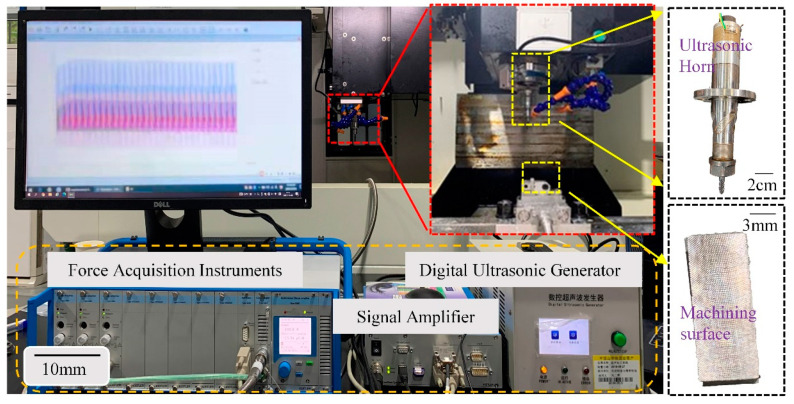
Longitudinal torsion ultrasonic-assisted milling test set.
